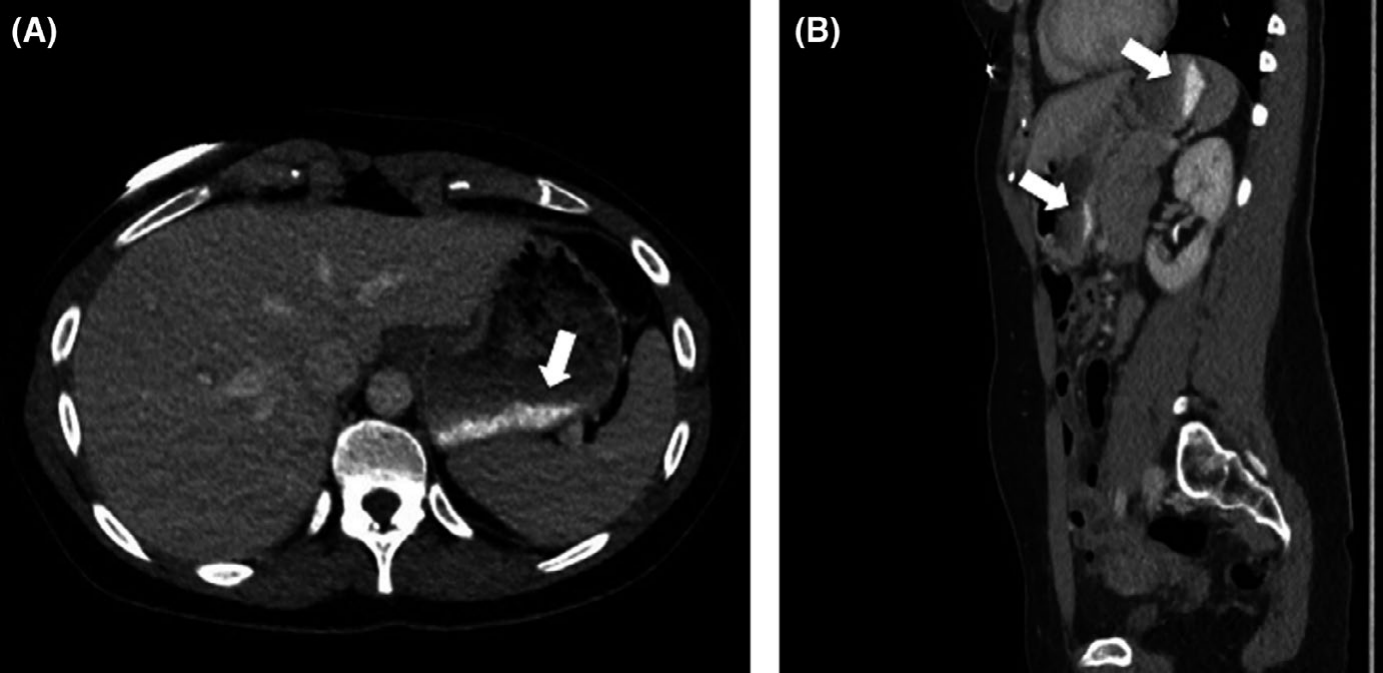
Contrast‐enhanced CT, axial (A) and sagittal (B) images. The bottom of the stomach is lined with an intensely dense content (270‐320 Hounsfield Units, white arrows), matching the density of iodine contrast
